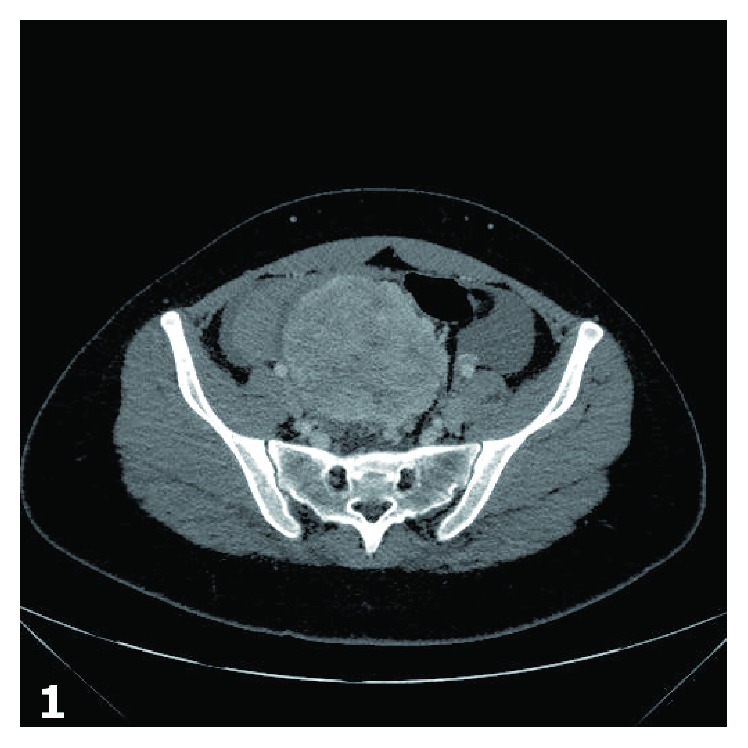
Computed tomography scan: There is a large irregular heterogeneous exophytic mass that appears to be arising from the right adnexa which intraoperatively arose from the serosa of the sigmoid colon.
